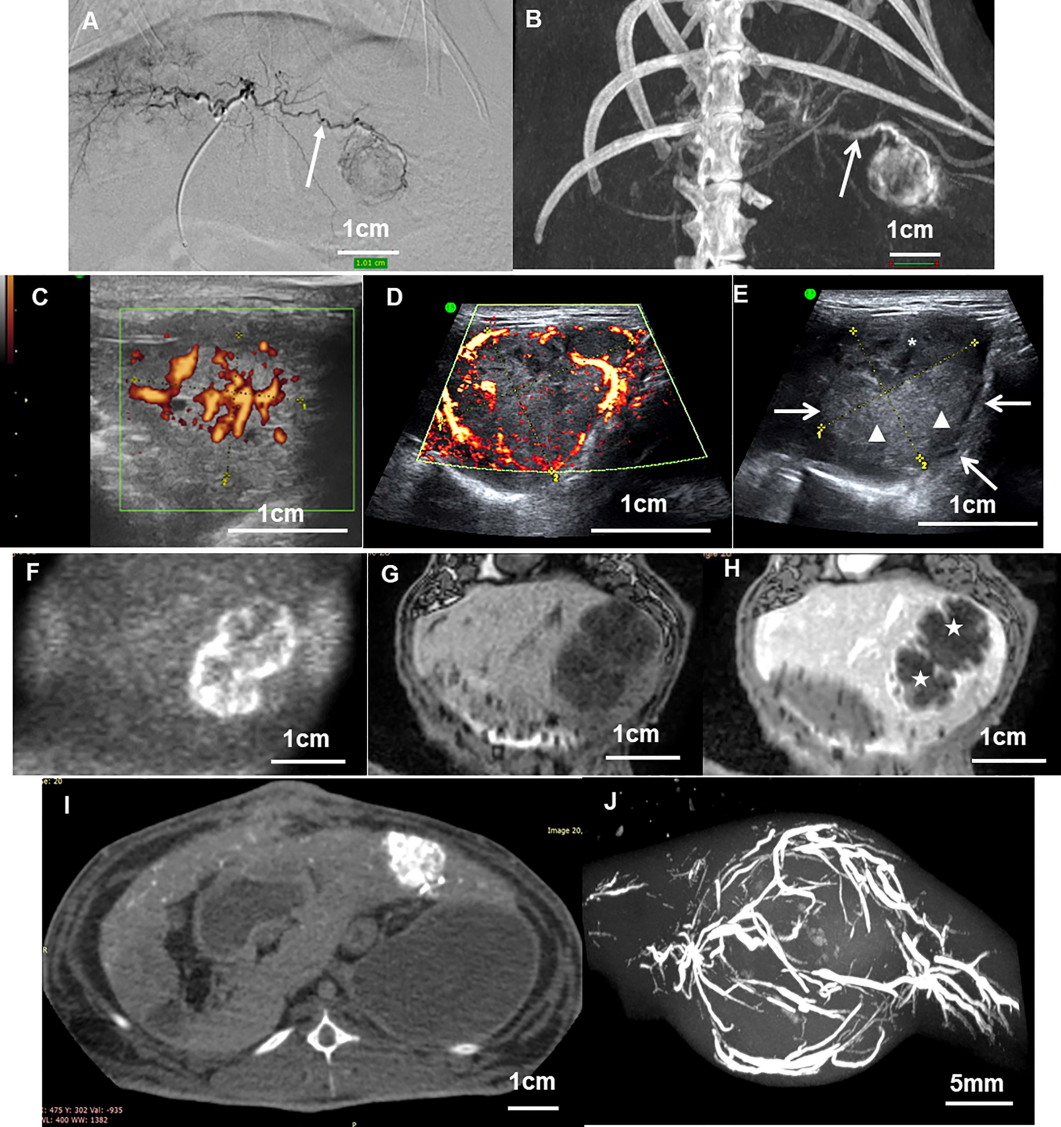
(A) Angiography and (B) cone beam CT acquisitions of a VX2 tumor after 13 days of tumor development showing the main tumor feeding artery (arrow). (C) Ultrasound image in Power Doppler mode of VX2 tumor at 13 days showing vessels inside the tumor core. (D) The same examination of the same tumor at 21 days showing vessels at the periphery of the nodule. (E) The same tumor in B mode gray scale showing the tumor as a heterogenous mass with hyperechogenic (arrow heads) and hypoechogenic (stars) areas that correspond to viable and necrotic areas respectively, and hypoechogenic aspect of the tumor boundaries (arrows). (F–H) Coronal MRI view of a 21-day tumor with diffusion weight imaging (F), T1-weighted before (G) and after (H) intravenous Dotarem contrast injection. The vessels are enhanced by gadolinium injection at the tumor boundaries while the necrotic core of the tumor remains unenhanced (stars). (I) Axial slice of a cone beam CT abdominal acquisition of a rabbit showing enhanced VX2 liver tumor after intra-arterial contrast injection. (J) Maximum intensity projection of a micro-CT acquisition of a VX2 tumor injected intra-arterially with radiopaque beads. Beads are seen with high attenuation in the vessels inside and around the nodule. All figure parts are from (16, 22).
